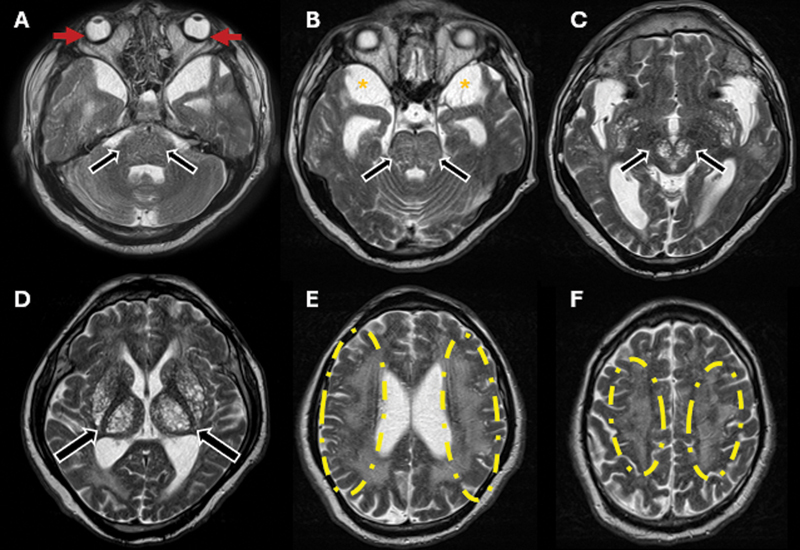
Brain magnetic resonance imaging (MRI) scan, axial T2-weighted images (
